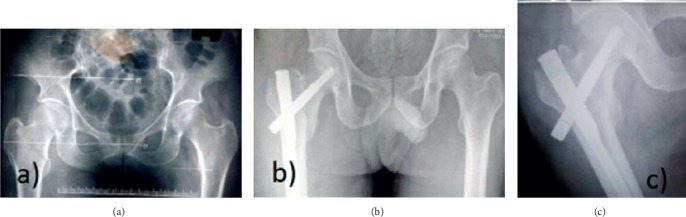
(a) Preoperative X-ray. (b) Immediate postoperative X-ray of PFNA-2 with lateral entry point showing lateral cortex impingement with gap at fracture site. (c) Follow-up at 6 months. Fracture union was seen at 6 months but with screw back out.
